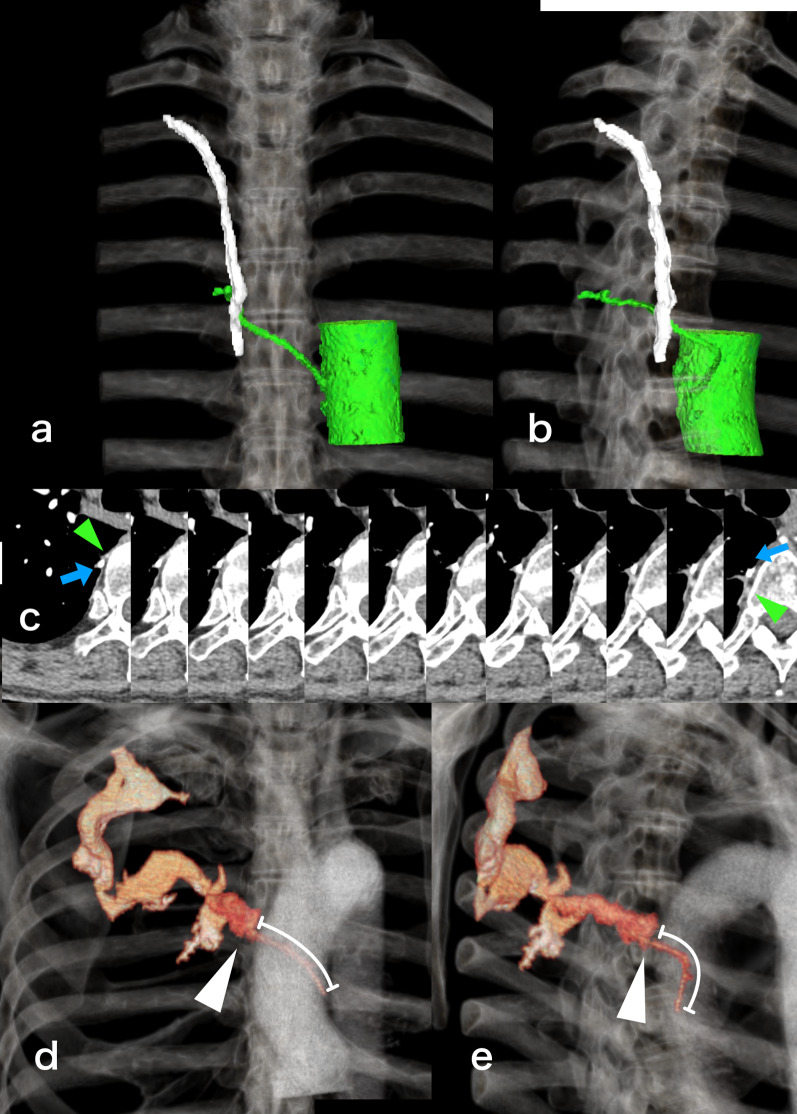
Relationship between the bleeding site of the fifth intercostal artery and the staple line. a Frontal view, b right anterior oblique view showing the relationship between the staple line (white) and the fifth intercostal artery (light green) reconstructed based on the CT scan from one month before hemothorax onset. c Sequential axial images showing the staple line (blue arrow) and the fifth intercostal artery originating from the aorta (light green arrowhead) crossing each other. d Frontal view, e right anterior oblique view of reconstructed contrast-enhanced CT scan at onset showing the disrupted fifth intercostal artery and the contrast medium leaking into the thoracic cavity. The intact part of the fifth intercostal artery indicated by white line, and the bleeding point indicated by white arrowhead. The staple line from the past pneumothorax surgery aligns with the fifth intercostal artery, and the bleeding point was exactly the same location. Despite a shock vital with BP 50/30 and HR 120, an approximate blood loss of 40 mL/min was measured. All of the contrast medium leaked out at the bleeding point, and the peripheral fifth intercostal artery beyond the bleeding point was not visualized
